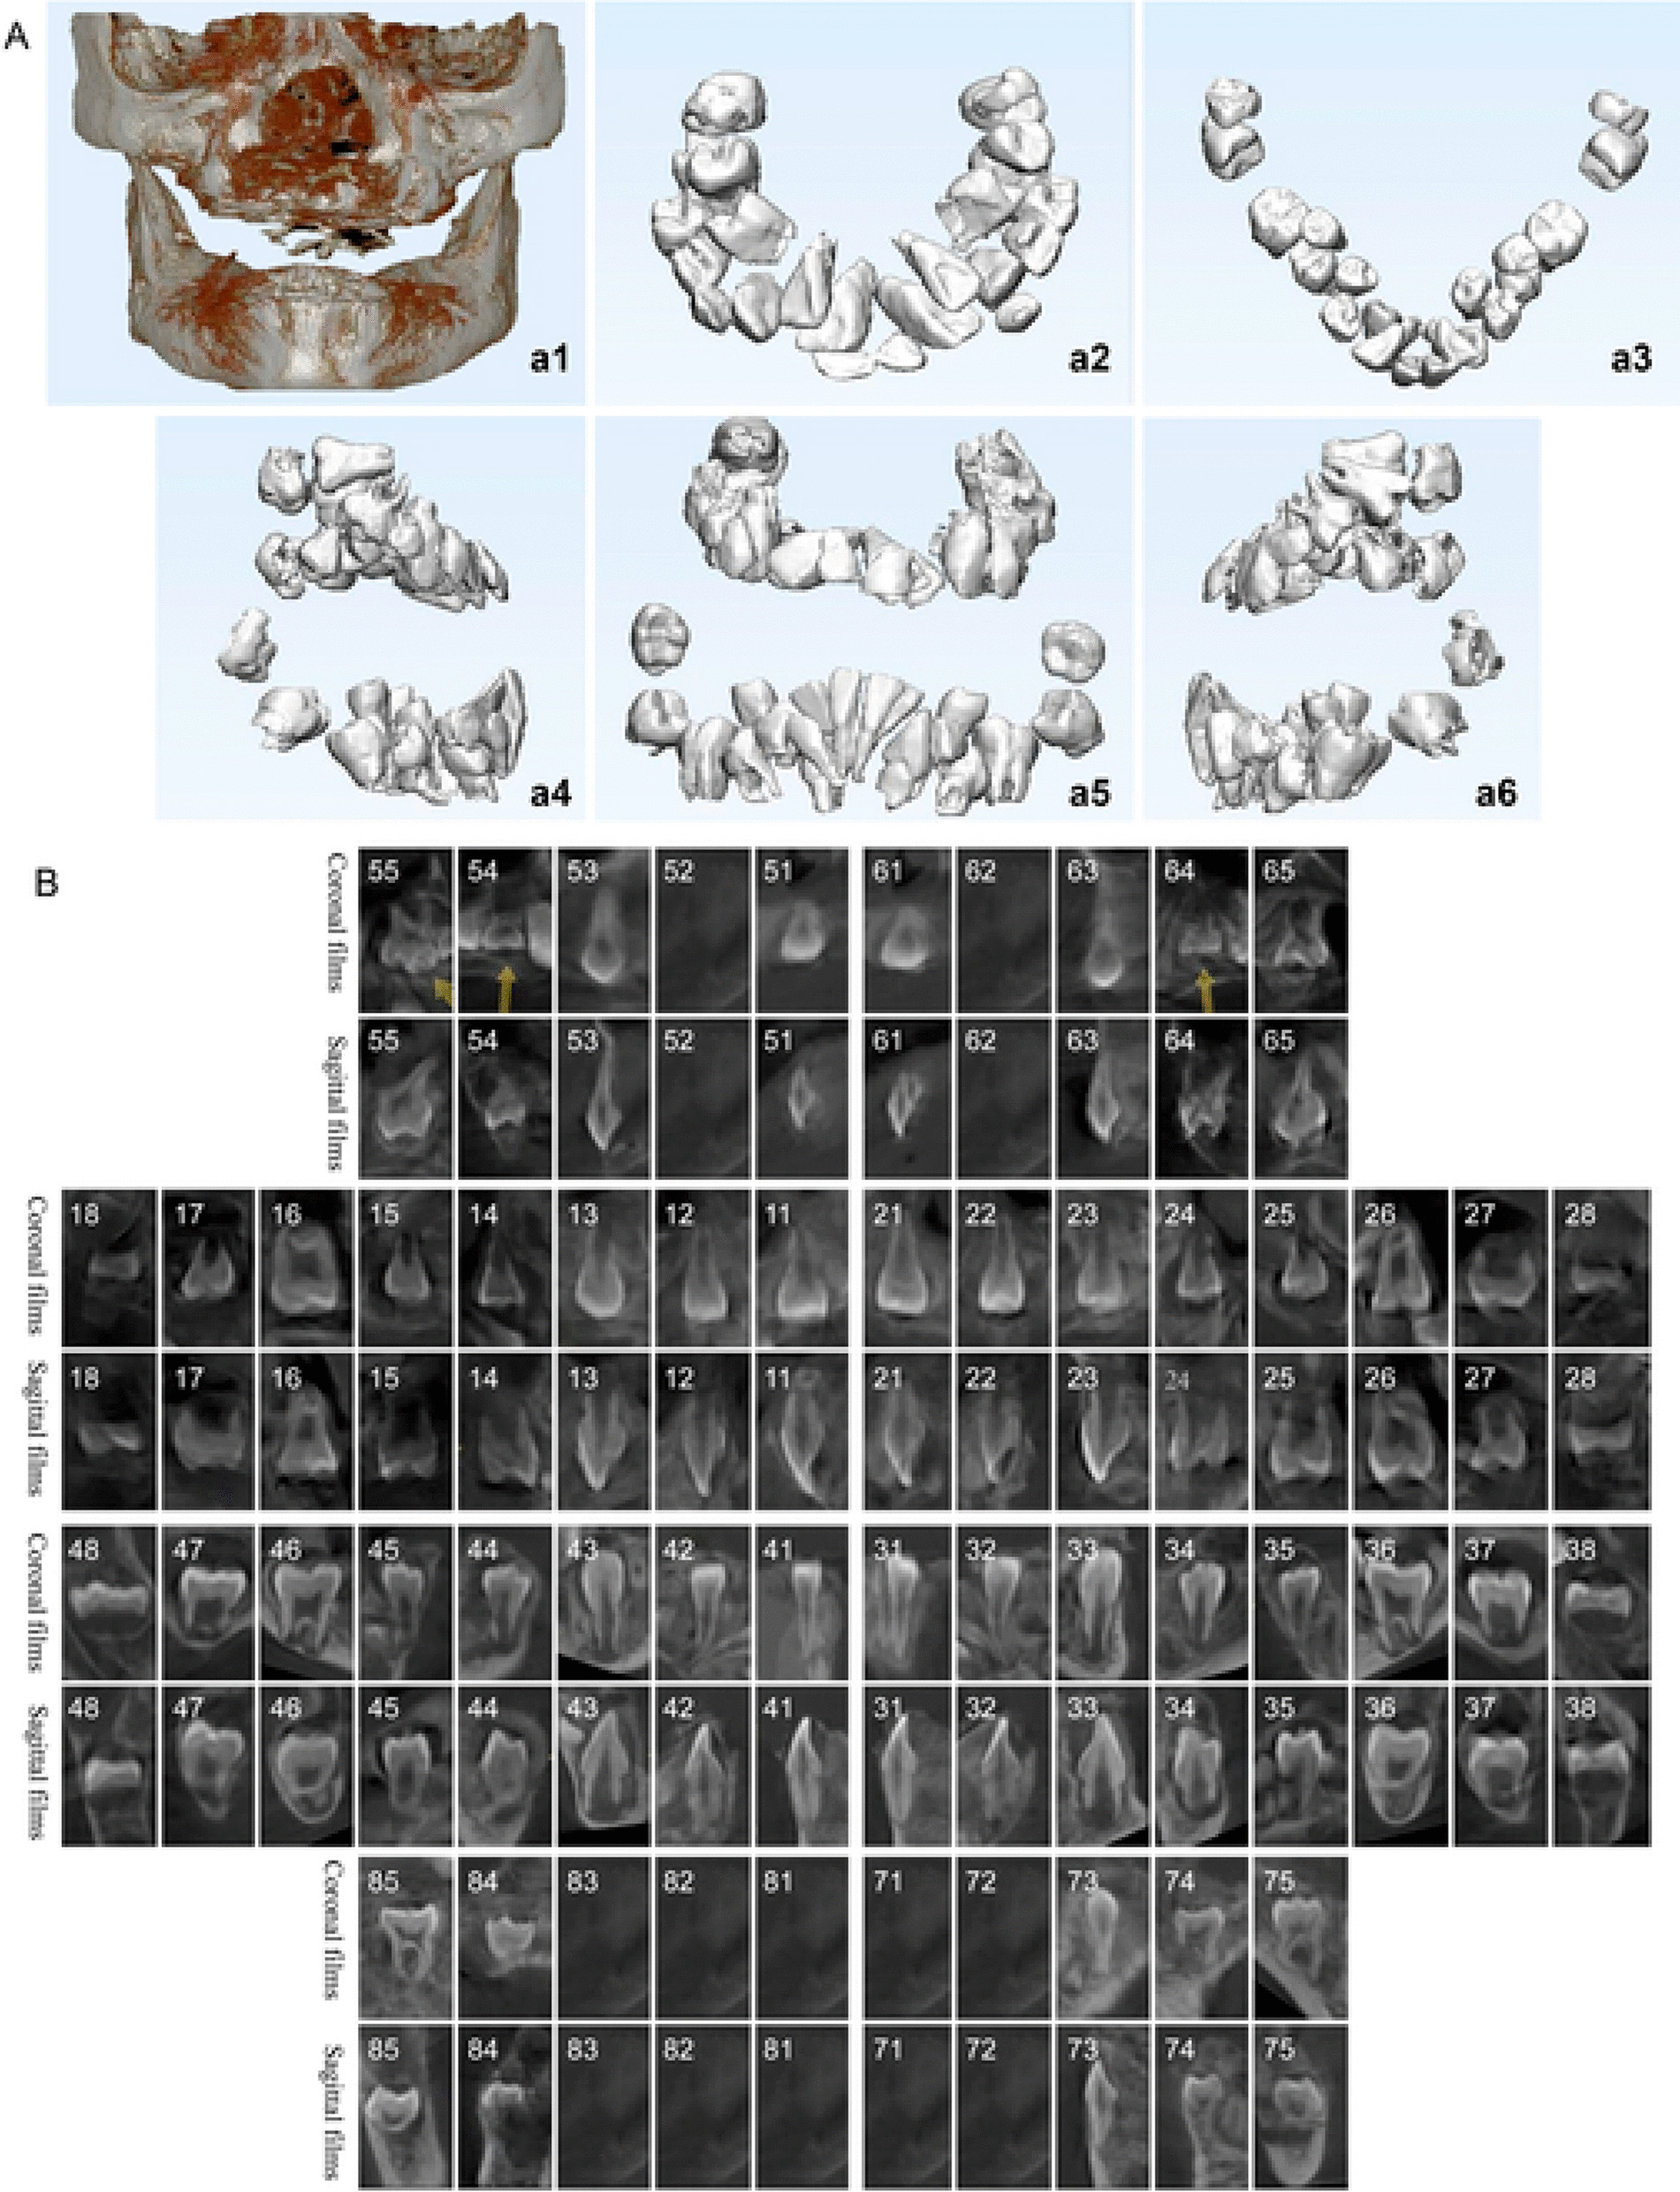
Cone-beam computed tomography (CBCT) images of his teeth with both three-dimensional reconstructed images (A) and two-dimensional images of the coronal and sagittal planes of each tooth (B)
